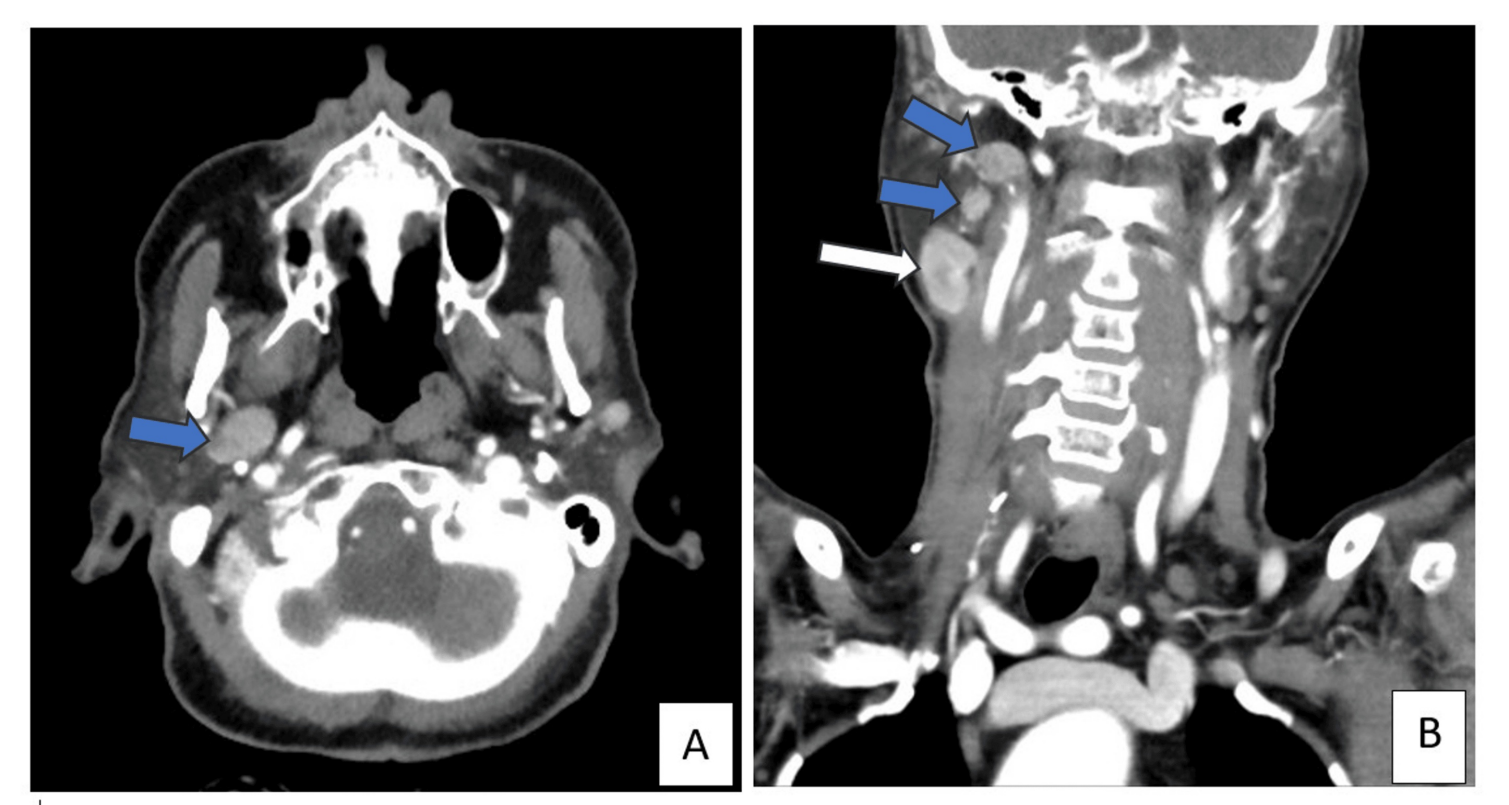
Contrastenhanced CT scan, (A) axial and (B) coronal views, of the neck at the level of parotid gland Few enhancing right cervical lymph nodes are noted (blue arrows) and a well-defined oval shaped hyper-enhancing lesion within the right parotid gland (Warthin’s tumour) (white arrow).
